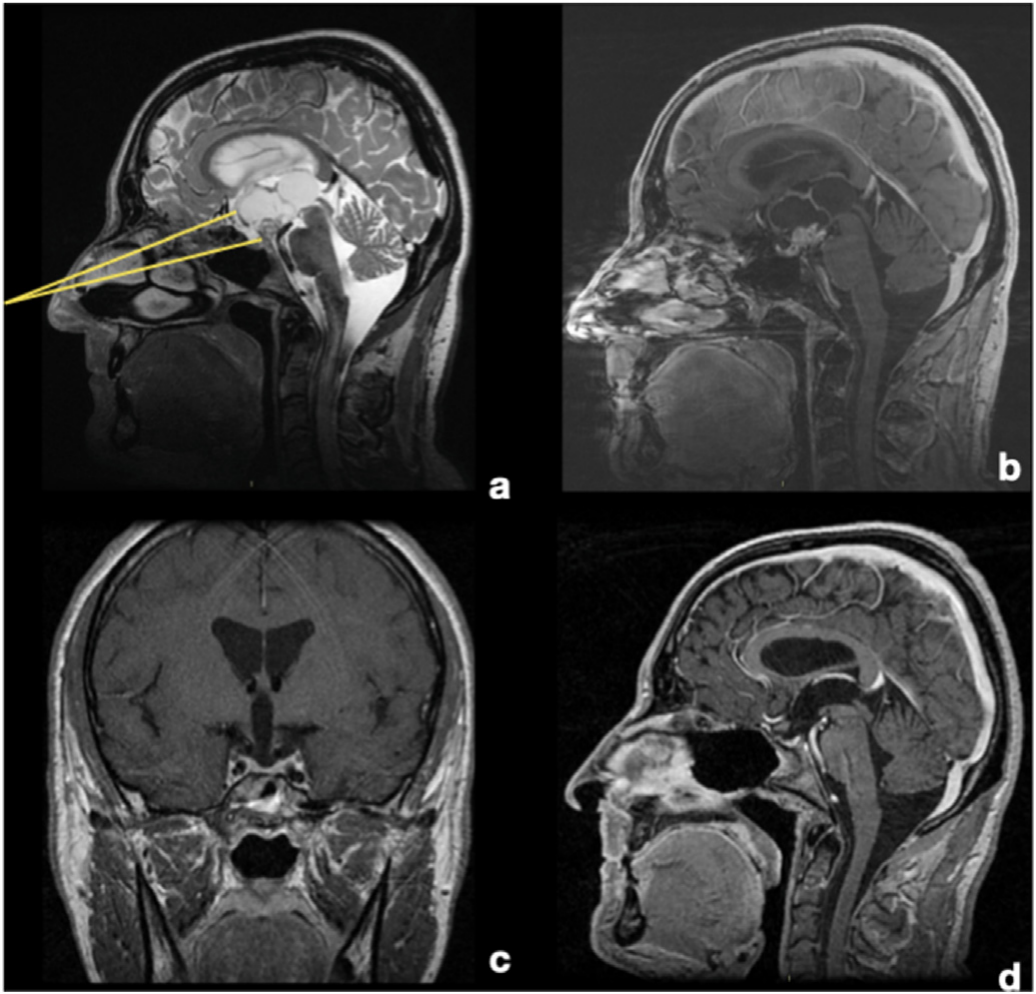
MRI T2-w and T1-w with gadolinium (sagittal and coronal slices). A and B. Pre-operative MRI demonstrating a partially cystic tumor invading the third ventricle. The suprasellar portion is mostly solid with some calcifications, while the intra-ventricular one has a mixed consistency, with a large cystic appearance. Pituitary stalk is not clearly recognizable, but it seems displaced posteriorly by the tumor Hypothalamic structures were displaced circumferentially around the tumor, while the optic chiasm was displaced antero-superiorly and no signs of hydrocephalus were present. The EEA was chosen because, as showed with yellow lines, it could give a direct and straight corridor to the tumor, without the interposition of any eloquent structures, as optic chiasm, stalk or hypothalamus. C and D. Post-operative MRI. A complete tumor removal has been achieved. The optic chiasm has been decompressed and it has recovered its normal location, while hypothalamic structures have been preserved. Because of the strict adherence between the tumor and the stalk, it has been resected during surgery. (For interpretation of the references to colour in this figure legend, the reader is referred to the Web version of this article.)
